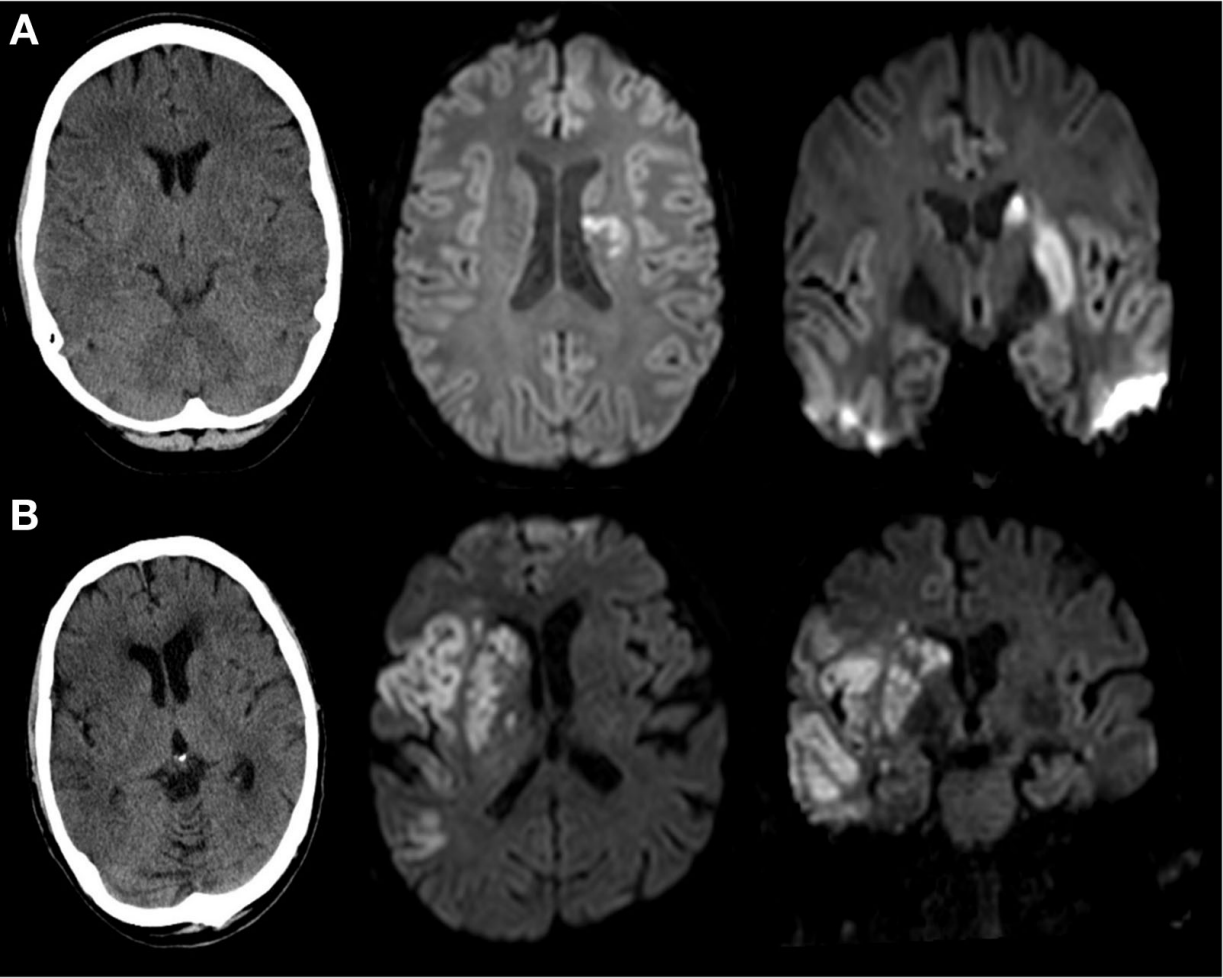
Imaging examples of isolated (iSCI) and non-isolated striatocapsular infarct (niSCI). Left column, non-contrast CT; middle column, axial diffusion-weighted imaging; right column, coronar diffusion-weighted imaging. (A) iSCI; (B) niSCI.
